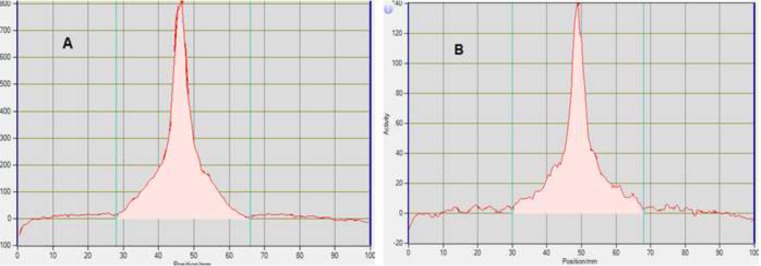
HPLC Chromatograms of ^18^FDG after synthesis (A) and 10 h later (B) at r.t.
